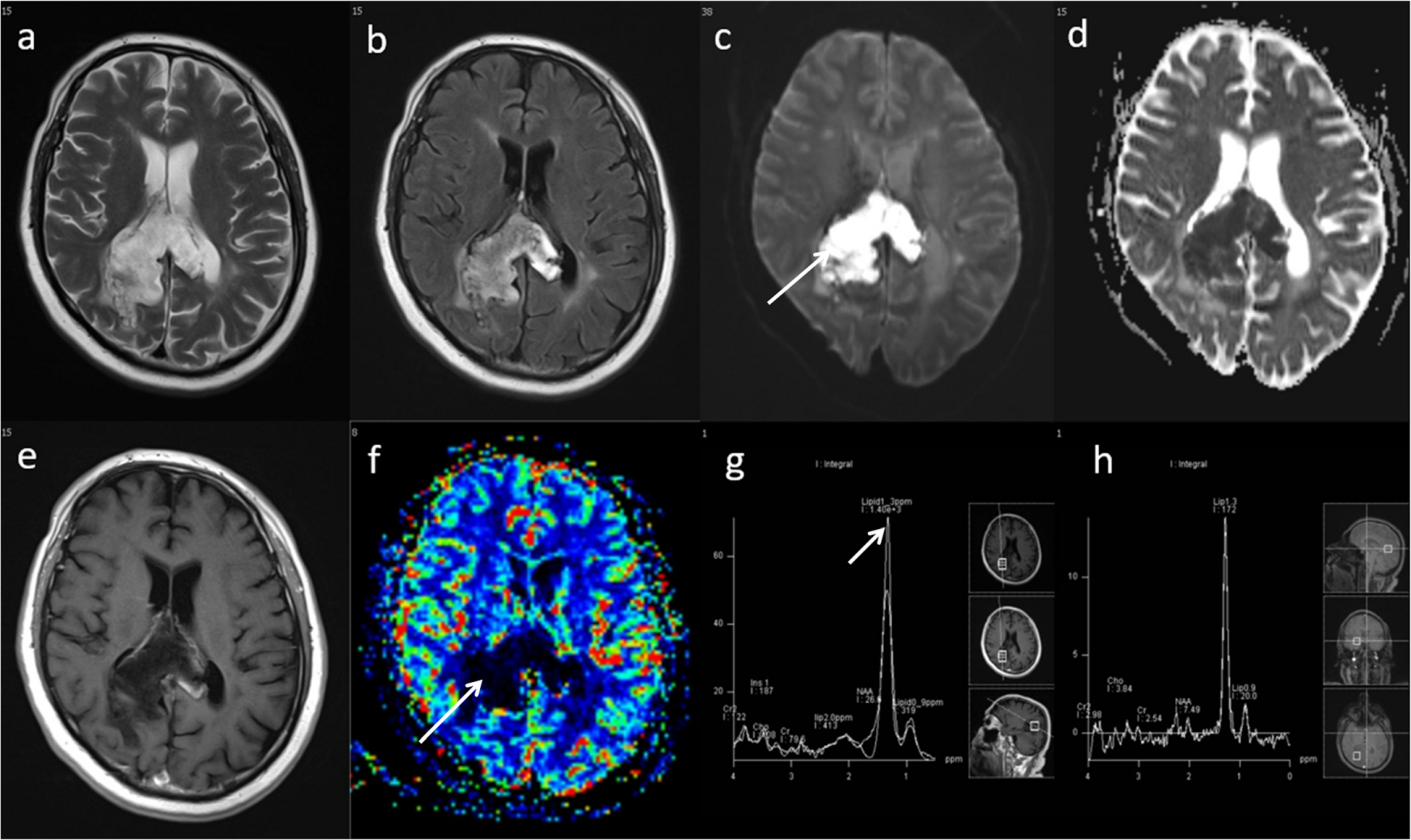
Epidermoid-like lesion of the corpus callosum. Conventional MRI: a, b, e T2W, FLAIR and post-contrast T1W sequences show a lesion involving the splenium of the corpus callosum and right parietal lobe. Multiparametric MRI: c, d DWI and ADC images show restricted diffusion (arrow). f Very low perfusion on PWI (arrow). g MRS shows very high lipid (1.3 ppm, arrow), without an increase in choline. In this case, appearances are not typical for high-grade glioma as there is low perfusion and no significant increase in choline, and it is not typical for lymphoma as there is no contrast enhancement or raised choline. Biopsy was consistent with epidermoid-like lesion with no evidence of tumour
